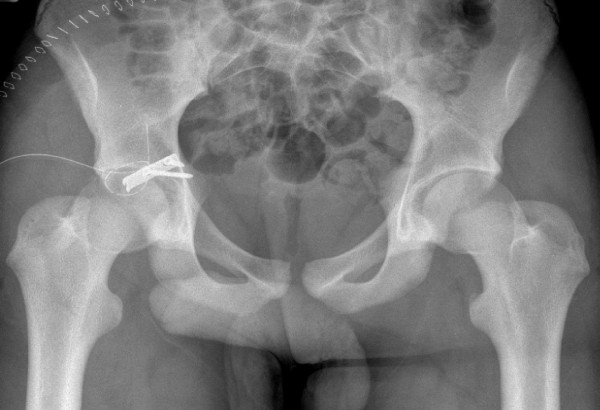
Postoperative X-ray (Case 1).
